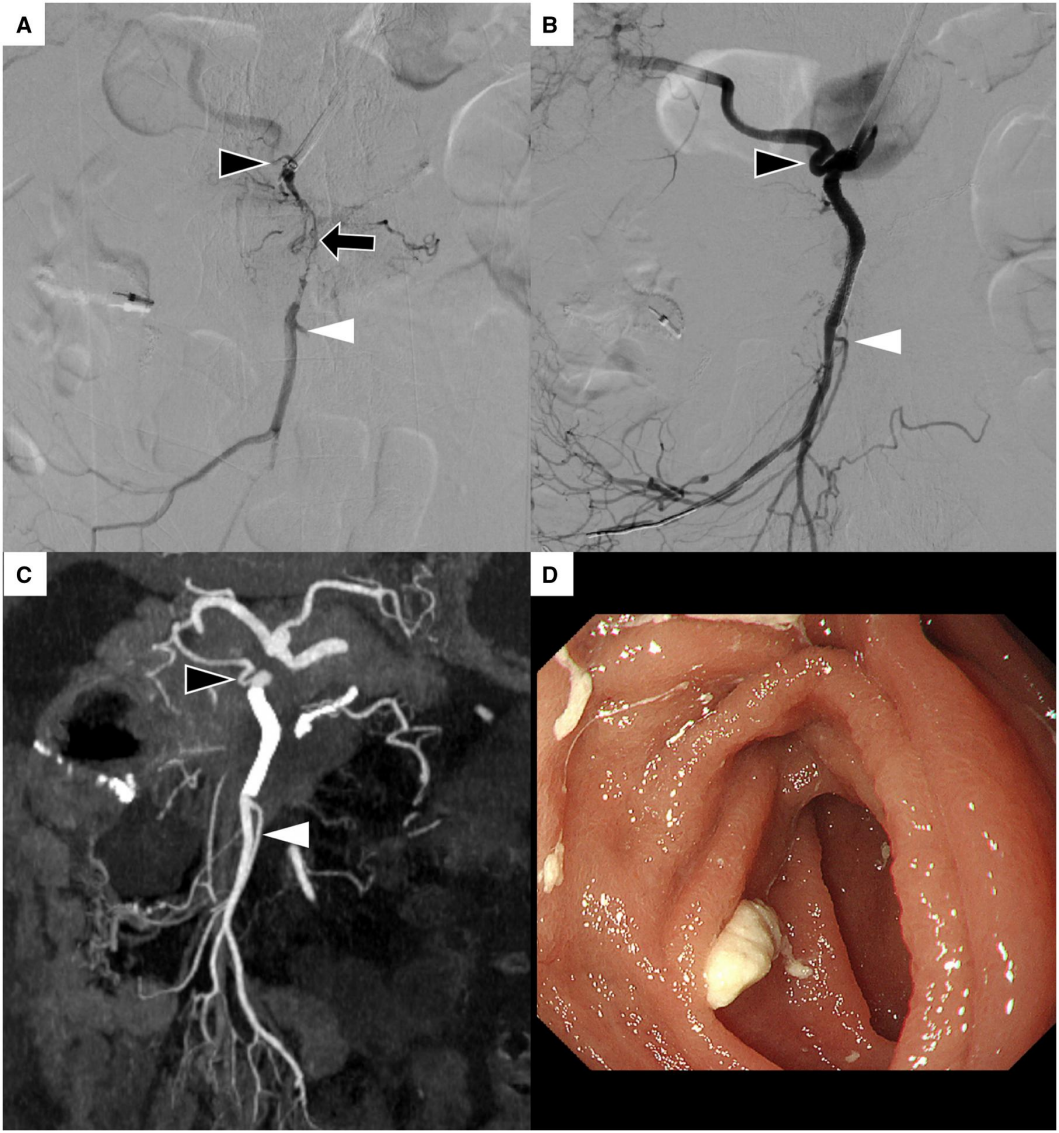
(A) Superior mesenteric artery (SMA) angiography shows severe and long stenosis (black arrow) at the area of tumour invasion. Right hepatic artery (black arrowhead) and ileal artery (white arrowhead) originate near stenosis. (B, C) SMA angiography and coronal contrast-enhanced computed tomography (CECT) images after stenting show stent patency and improvement of intestinal blood flow. Right hepatic artery (black arrowhead) and ileal artery (white arrowhead) are patent without stent covering. (D) Endoscopic image after SMA stent placement shows the disappearance of multiple ulcers, mucosal erosions and intestinal haemorrhage caused by bowel ischaemia.
